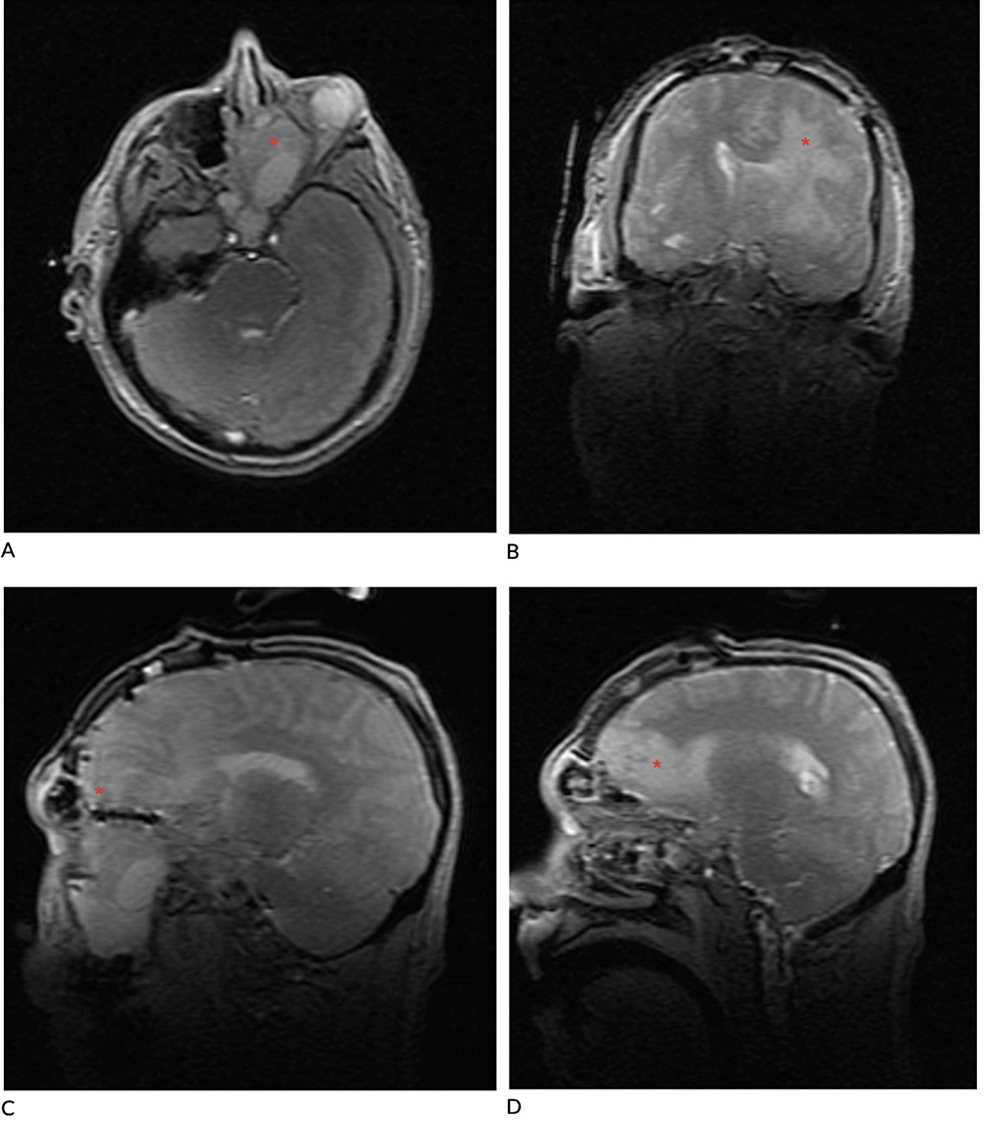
Repeat MRI status post-craniotomy resection of mass. A: axial MRI showing the extra-orbital area of resection of sinonasal mass, indicated by the red asterisk; B: axial MRI showing varying areas of vasogenic edema status post craniotomy, indicated by the red asterisk; C-D: sagittal MRI showing hyperattenuating areas of vasogenic edema consistent with normal changes after complete tumor resection, indicated by the red asterisk.
